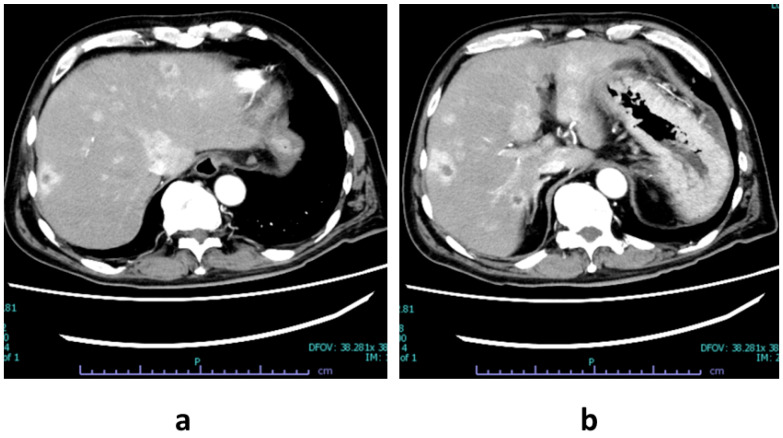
Contrast-enhanced abdominal CT (arterial phase) showing multiple hypovascular liver metastases from recurrent pancreatic ductal adenocarcinoma. (a) Axial image demonstrating multiple low-attenuation nodules with peripheral rim enhancement in both hepatic lobes. (b) Another slice showing a dominant hypovascular lesion in the right hepatic lobe, also exhibiting a ring-like enhancement pattern, consistent with metastatic disease.
